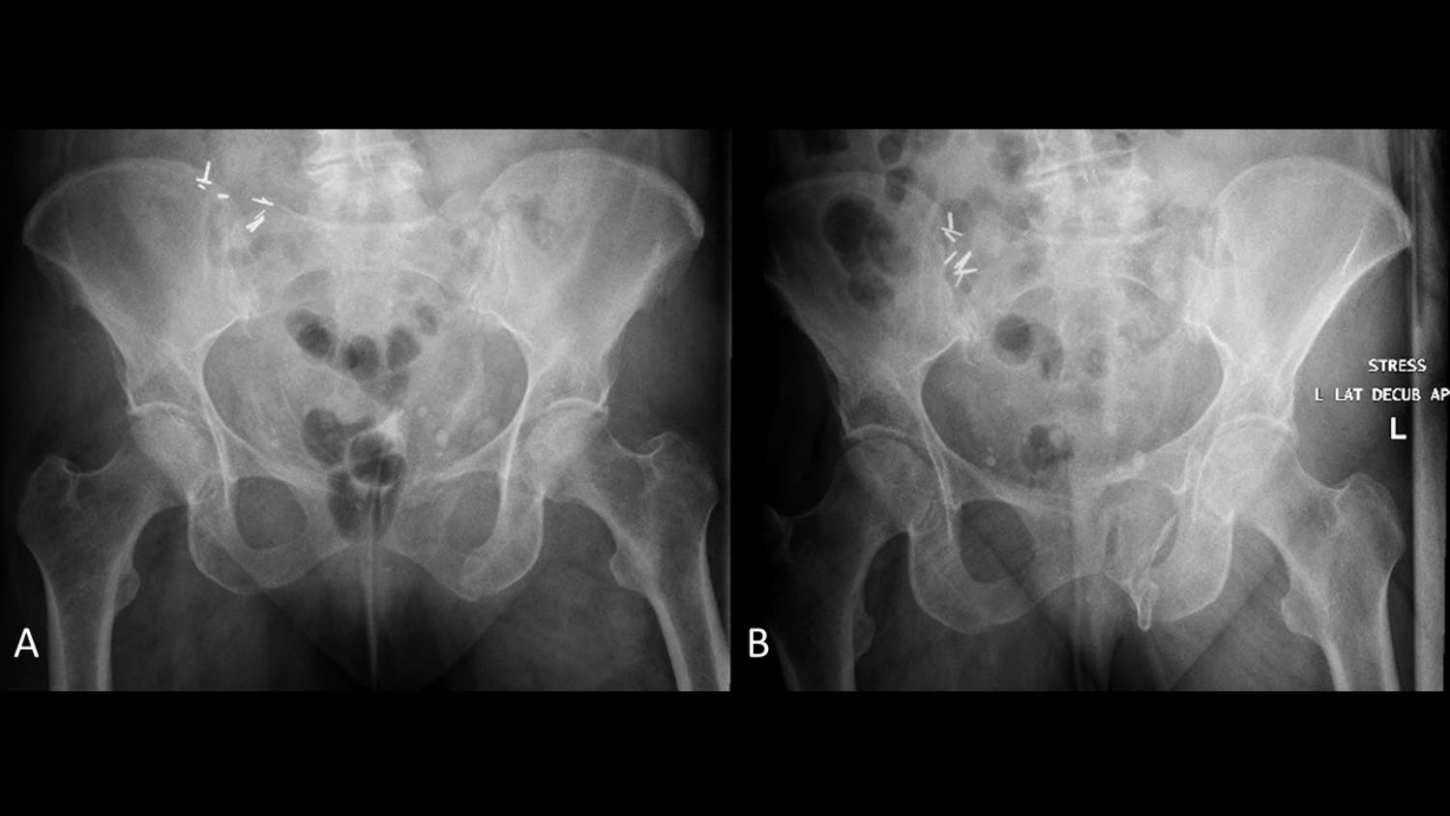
(A) AP pelvis radiograph and (B) lateral stress radiograph of the same patient from Fig. 1 redemonstrating the gross occult instability
